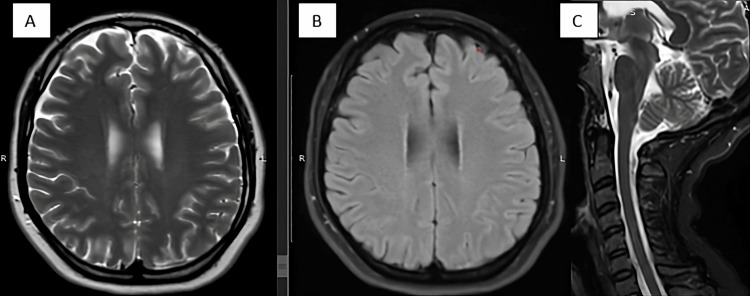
Initial brain and spine MRI A, B: Axial T2 and axial FLAIR show no structural injuries. C: STIR – cervical spine cord shows no lesions. MRI, magnetic resonance imaging; FLAIR, fluid-attenuated inversion recovery; STIR, short tau inversion recovery.
